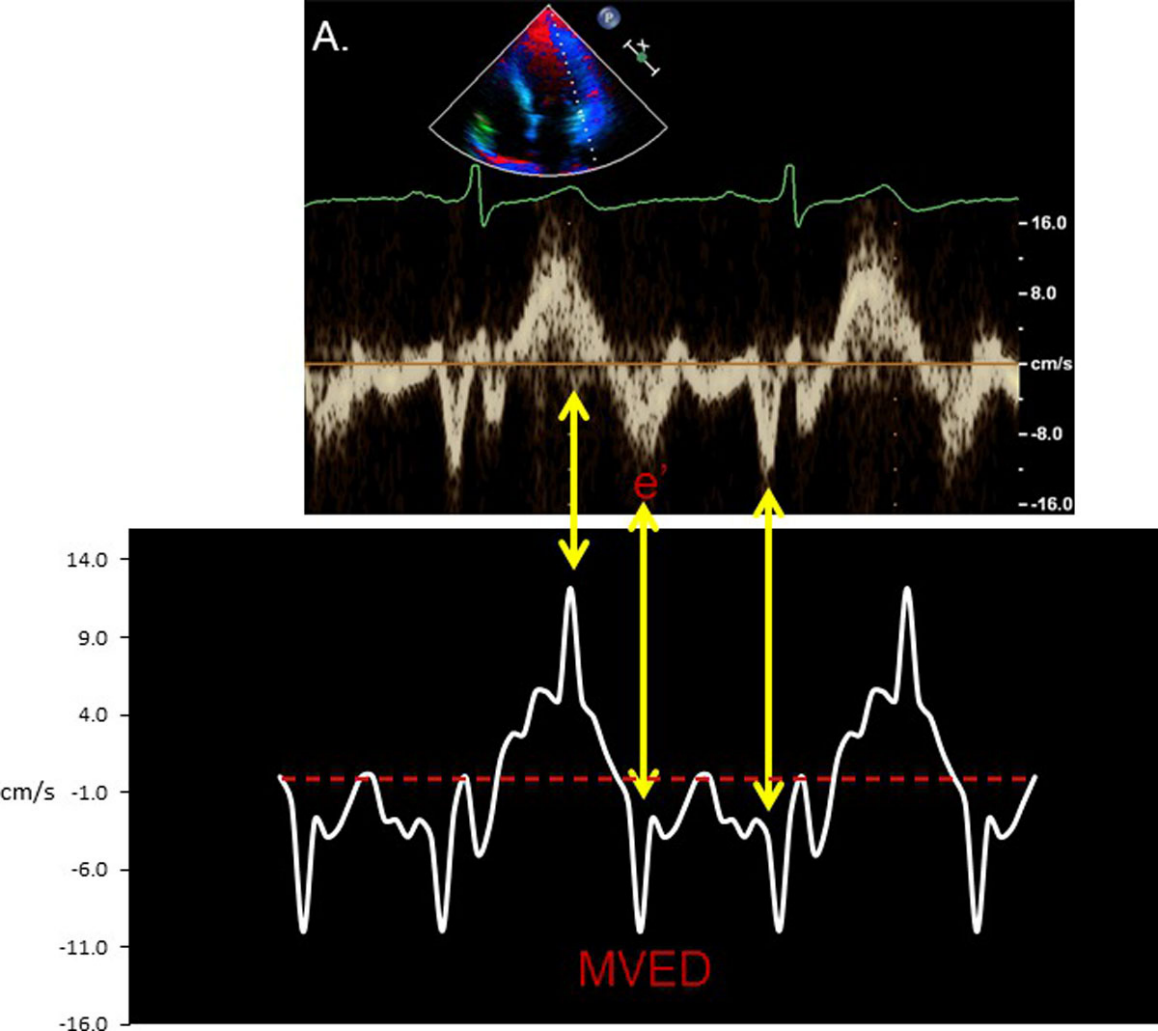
TDE Mitral Annulus and CMR AVJ Velocity Correlation. A. TDE demonstrates s', e' and a' waves corresponding to mitral annular velocities during systole, early diastole and with atrial contraction, respectively for a representative patient. B. Corresponding CMR-derived AVJ velocity-versus-time curve. Bidirectional arrows demonstrate corresponding mitral annular velocities during various phases of the cardiac cycle. AVJ = atrioventricular junction; MVED = mitral velocity early diastole; TDE = tissue Doppler echocardiography.
